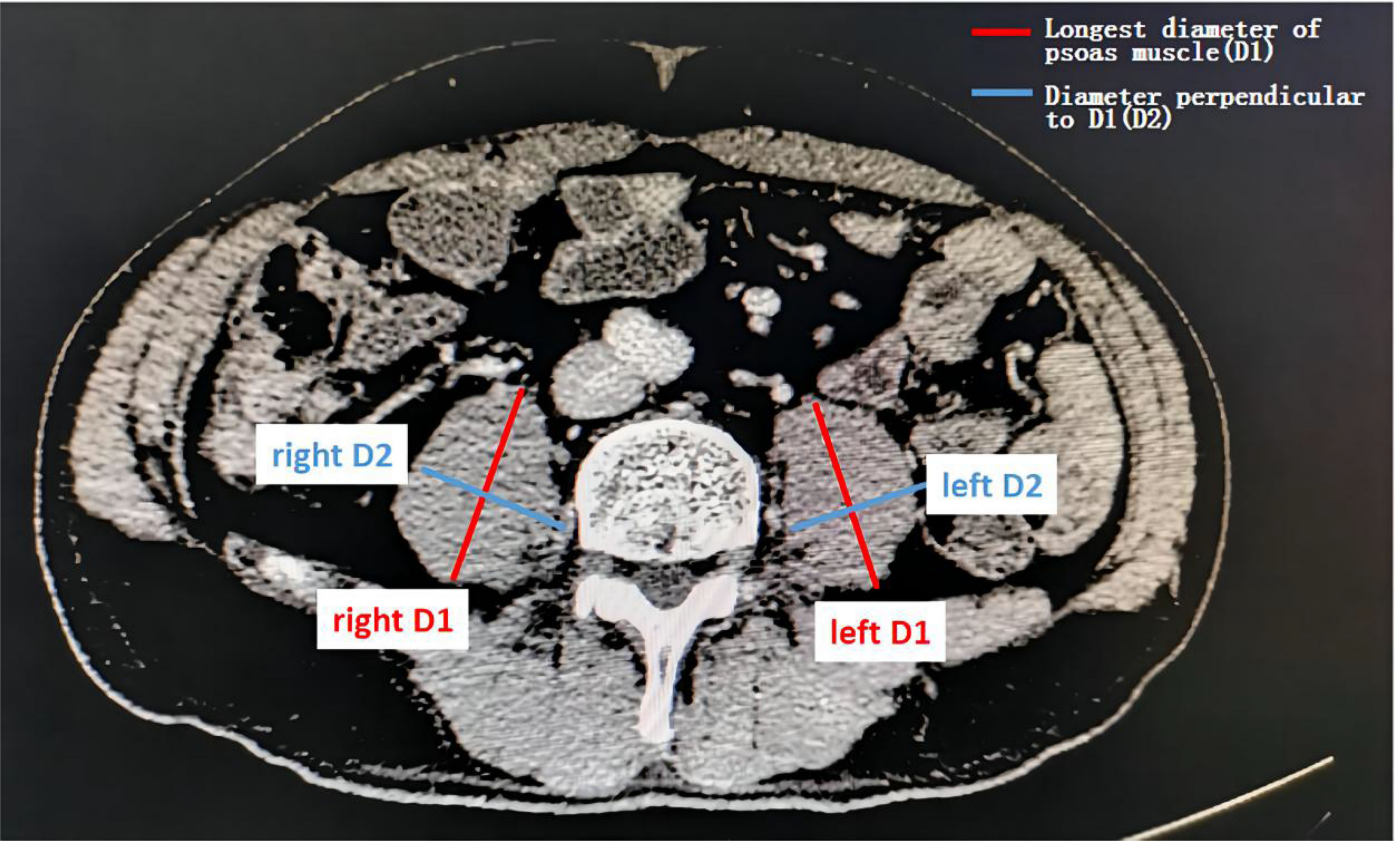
Assessment of the psoas muscle index (PMI). Sarcopenia was assessed by measuring the longest diameter (D1) and the perpendicular diameter (D2) of the right (ri) and left (le) psoas muscle on an axial computed tomography (CT) scan in the same plane and normalizing it for the patients’ height squared. This value is referred to as the psoas muscle index (PMI).
